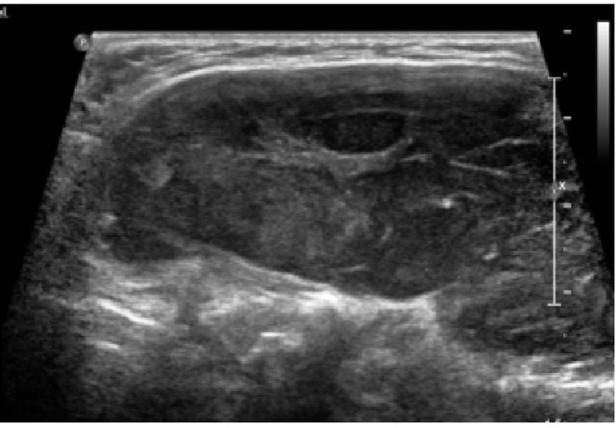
Thyroid ultrasound image of a nodule subsequently diagnosed as lymphoma. Image shows diffuse enlargement of the thyroid gland, characterized by a markedly heterogeneous and hypoechoic echotexture with smooth margins. No discrete thyroid nodule can be clearly identified.
